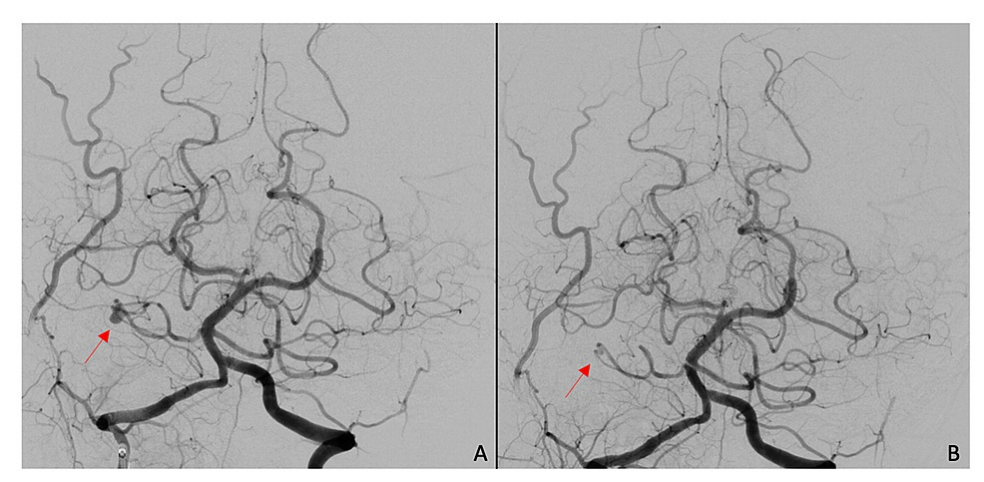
CT angiogram (A) pre-embolization and (B) post-embolization, demonstrating the absence of filling of right AICA AICA: anterior inferior cerebellar artery; CT: computed tomography
